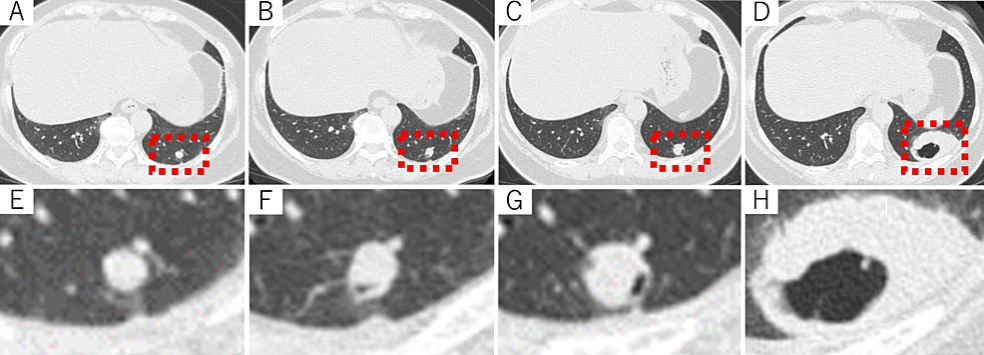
Pulmonary metastasis associated with gradually progressive intra-tumoral cavitation and subsequent anaplastic transformation (A-D) Chest CT scans taken (A) 10 years, (B) 11 years, (C) 12 years, and (D) 13 years after pulmonary metastasis diagnosis. (E-H) Enlarged views of the pulmonary metastasis are shown in Figures 2A, 2B, 2C, and 2D, respectively.
